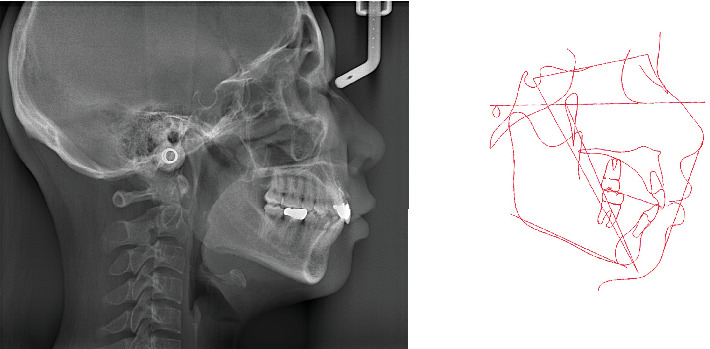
Posttreatment lateral cephalometric radiograph and tracing.
